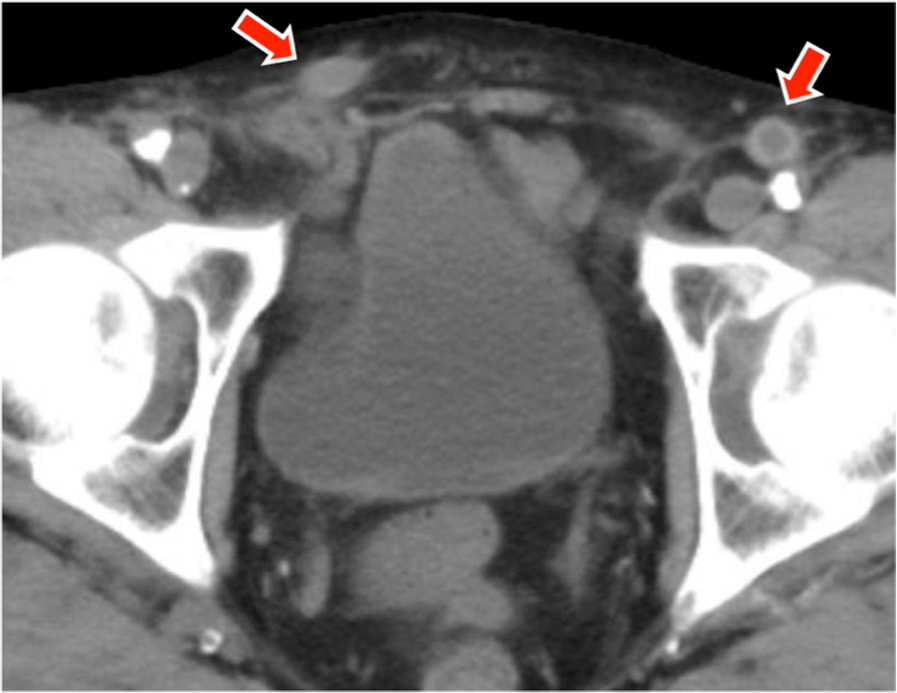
Non-enhanced abdominal CT scan (axial) showing the vascular prosthesis bilaterally (*arrow*), one on the *right* side positioned ventrally in the right inguinal canal
